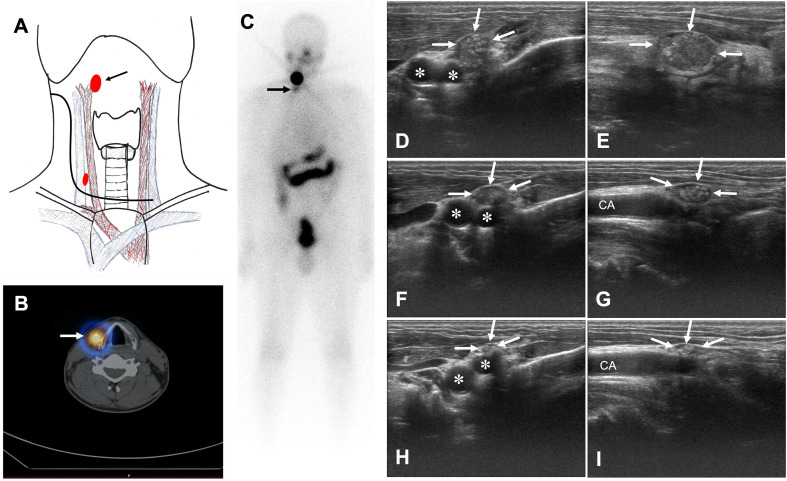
A 39-year-old man with ATA-2025 high-risk PTC (pT2N1bM0), underwent total thyroidectomy with central and right lateral lymph node dissection. Neck mapping at 6^th^ week showed residual metastatic lymph nodes (A), including a 1.5-cm node (black arrow) and 0.9-cm node in the right lateral cervical chain. After 5550 MBq RAI, SPECT/CT (B) showed intense focal uptake in the dominant node (white arrow), and WBS (C) showed discernible uptake in the smaller node inferior to the dominant lesion (black arrow). Pretreatment US of the dominant node (white arrows) demonstrated thyroidization and punctate echogenic foci [(D), axial; (E), longitudinal]. Marked regression was seen 4 months after RAI [(F), axial; (G), longitudinal], and the node was barely visible at 1 year [(H), axial; (I), longitudinal]. The patient has remained in the excellent response category since August 2020. ✽, external and internal branches of the common carotid artery; CA, common carotid artery.
